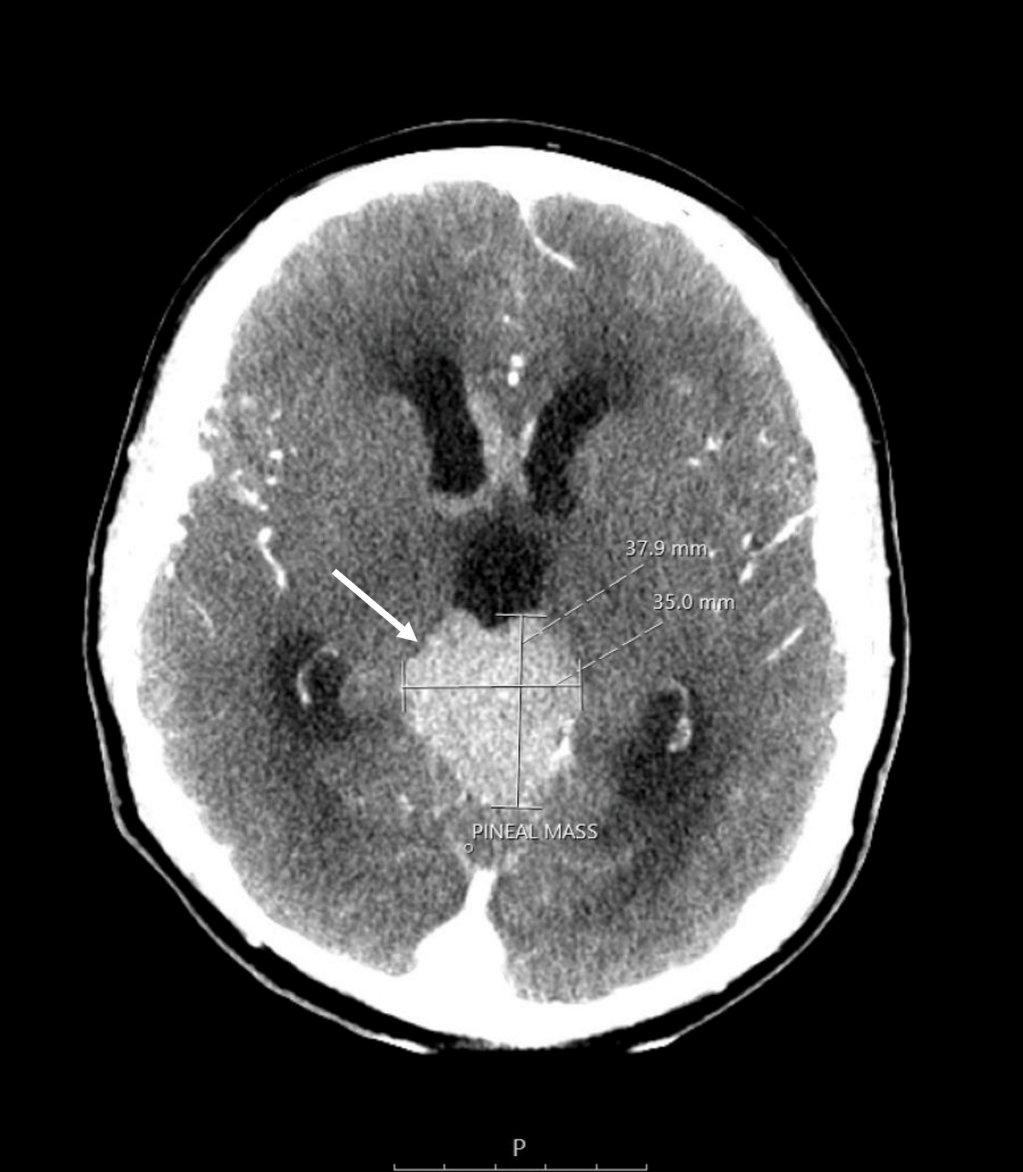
CT of the pituitary with contrast. White arrow: mass lesion in the pineal region.
